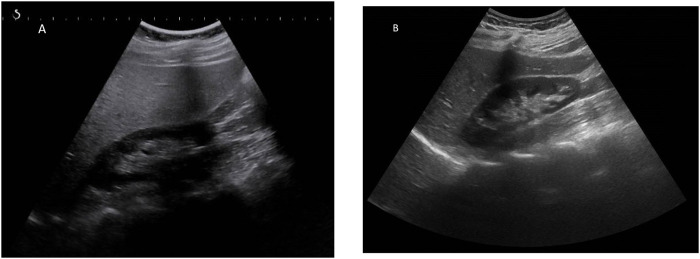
Ultrasonographic features seen in both GlyH and liver steatosis. Caption: (A) A greyscale ultrasound image of the liver parenchyma compared to the kidney will show hyperechogenicity of the liver in both GlyH and fatty liver disease, as seen in this 40-year old patient with T1D and NAFLD from our clinic. (B) In a normal liver, the echogenicity of liver and kidney parenchyma is similar, as seen in this 42- year old patient with T1D from our clinic.
